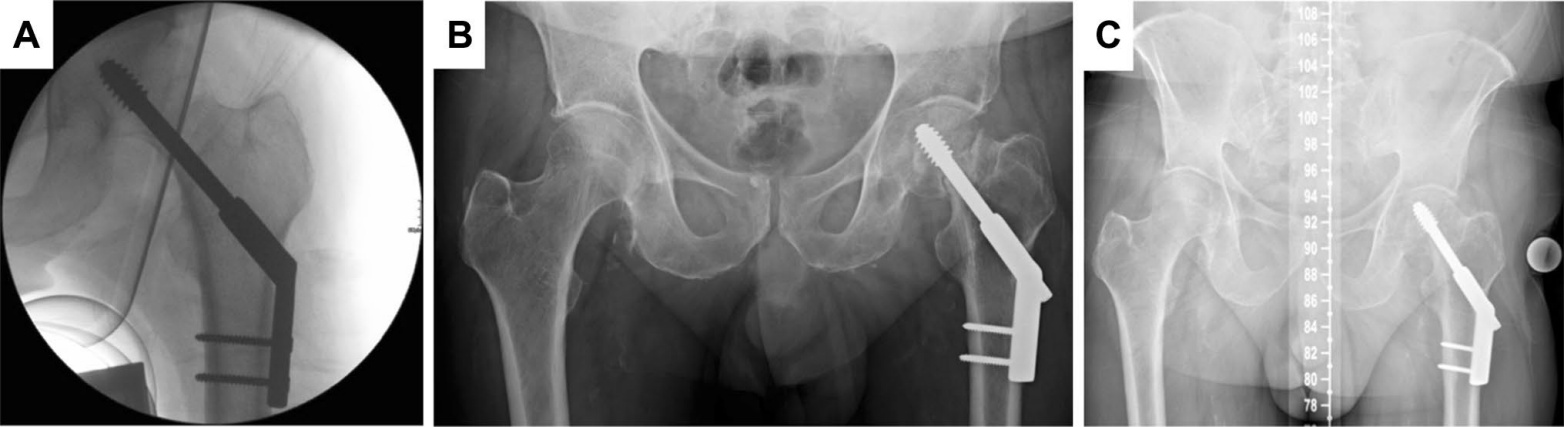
Patient (58 years) with medial femoral neck fracture and treatment with dynamic hip screw (DHS). a Intraoperative fluoroscopic image. b Follow-up x-ray 1 year after surgery. c Invited follow-up 6 years after surgery with leg length discrepancy (LLD) of 16 mm
